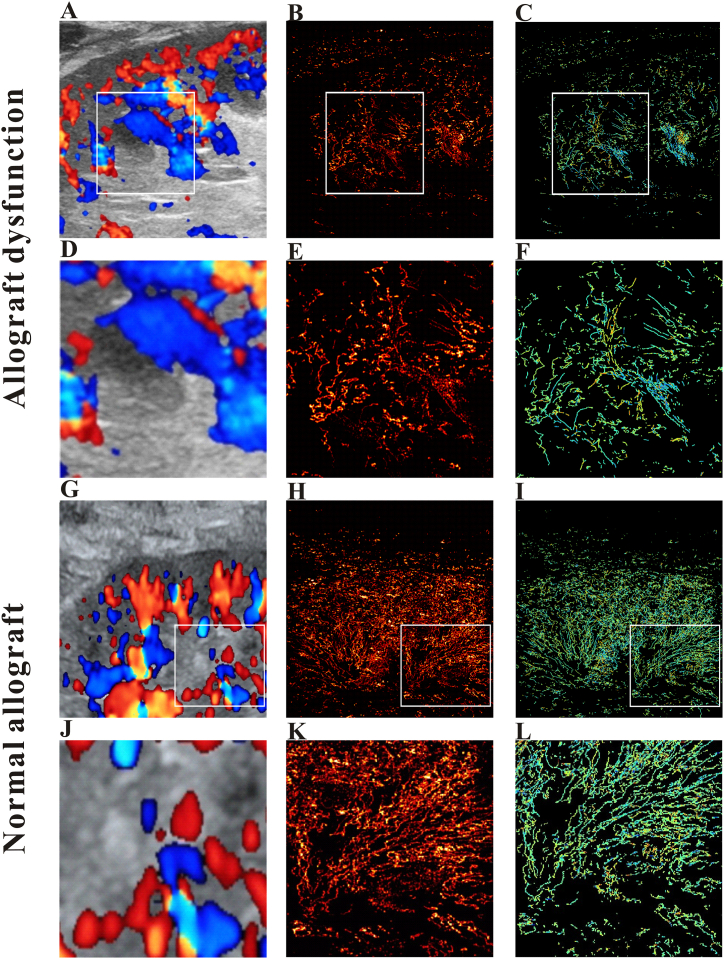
Ultrasound images of allograft dysfunction and normal allograft respectively. The color-doppler flow image (A), super-resolution image (B), super-resolved velocity image (C), and the zoomed-in sections indicated as the white box of the color-doppler flow image (D), super-resolution image (E), super-resolved velocity image (F) for allograft dysfunction, respectively. The color-doppler flow image (G), super-resolution image (H), super-resolved velocity image (I), and the zoomed-in sections indicated as the white box of the color-doppler flow image (J), super-resolution image (K), super-resolved velocity image (L) for normal allograft, respectively.
